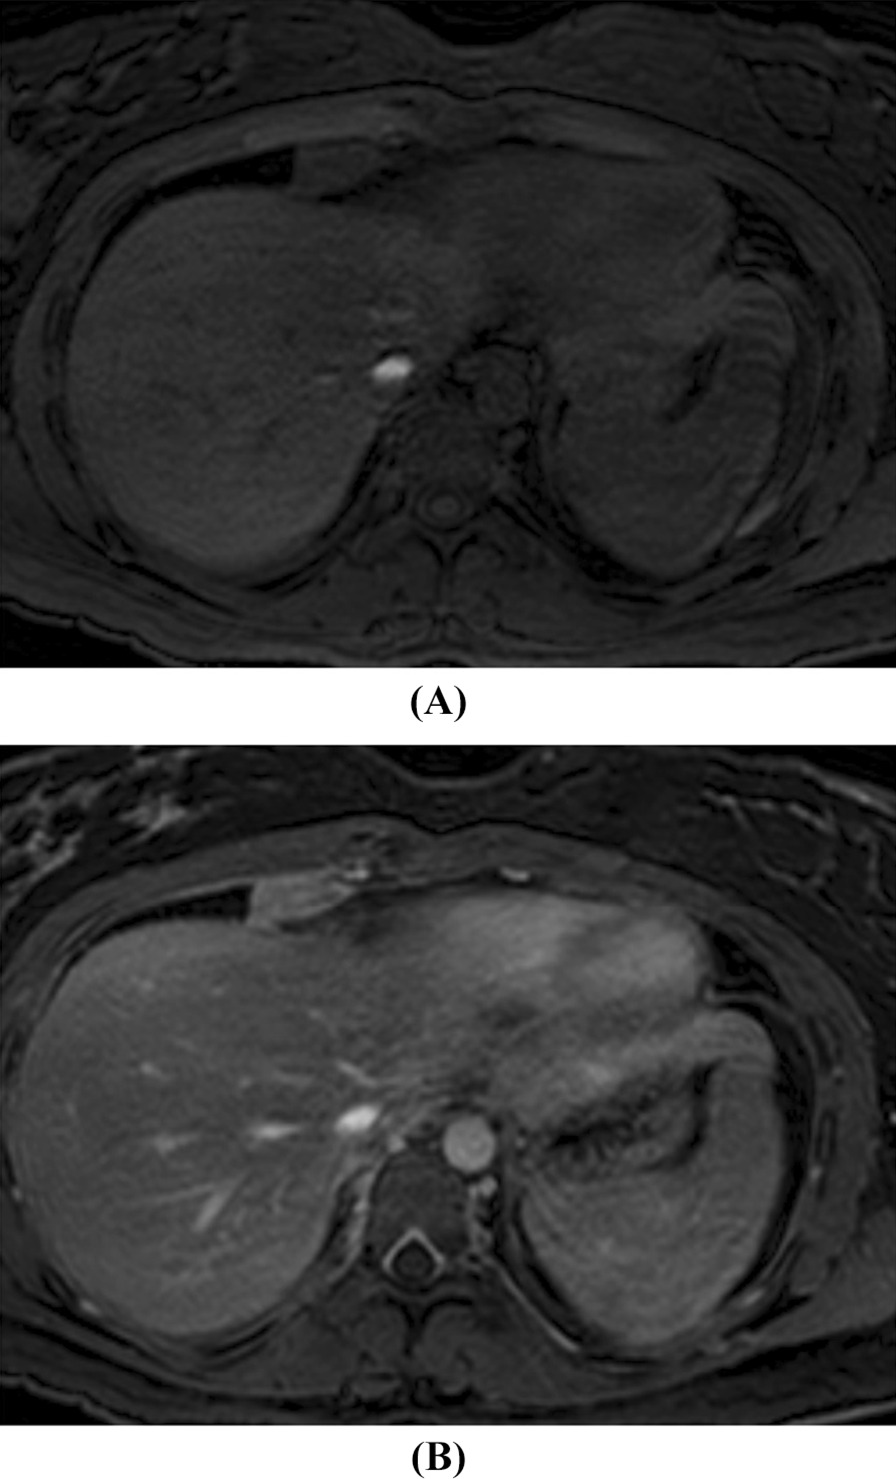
A Pre-contrast axial T1-weighted and B post-contrast axial T1-weighted MR images of the chest demonstrate a hypointense, avidly enhancing pleural based mass most consistent with a solitary fibrous tumor of the pleura
